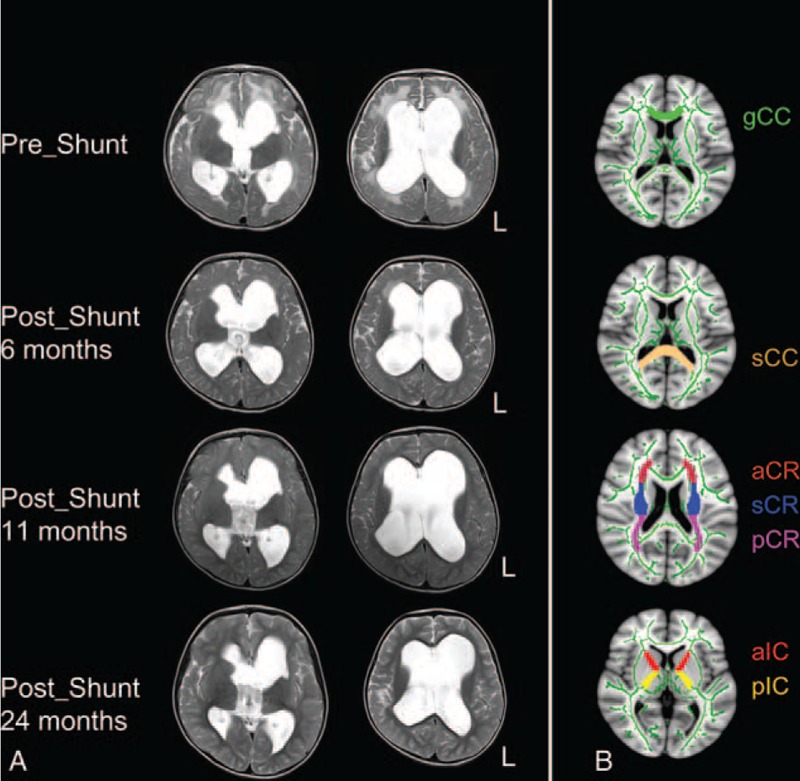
Patient's T2-weighted images and the location of the ROIs.
